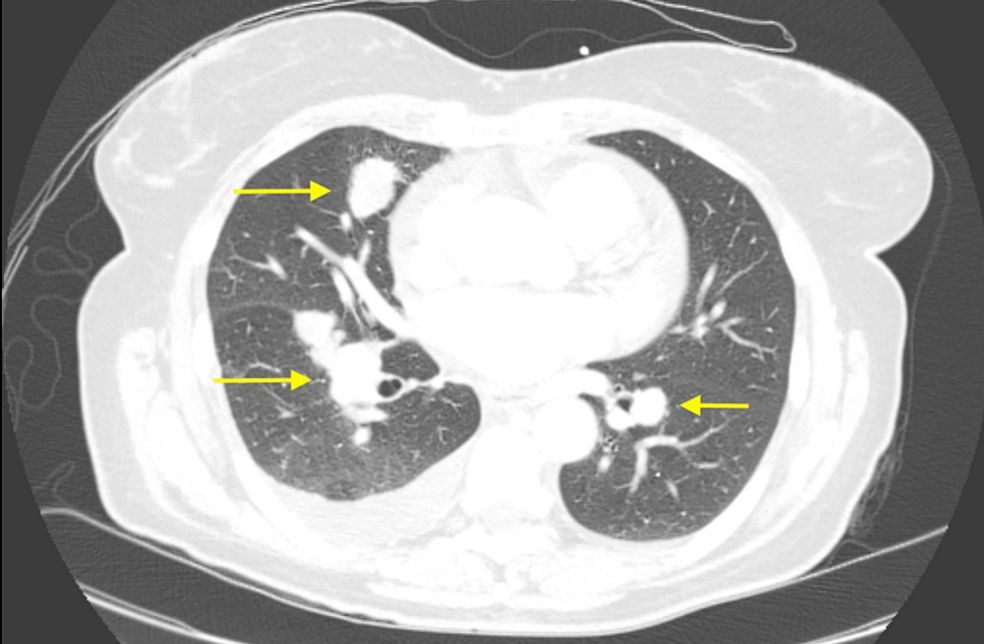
December 2022 CT chest with multiple mass-like consolidations. The yellow arrows point to the consolidations.
